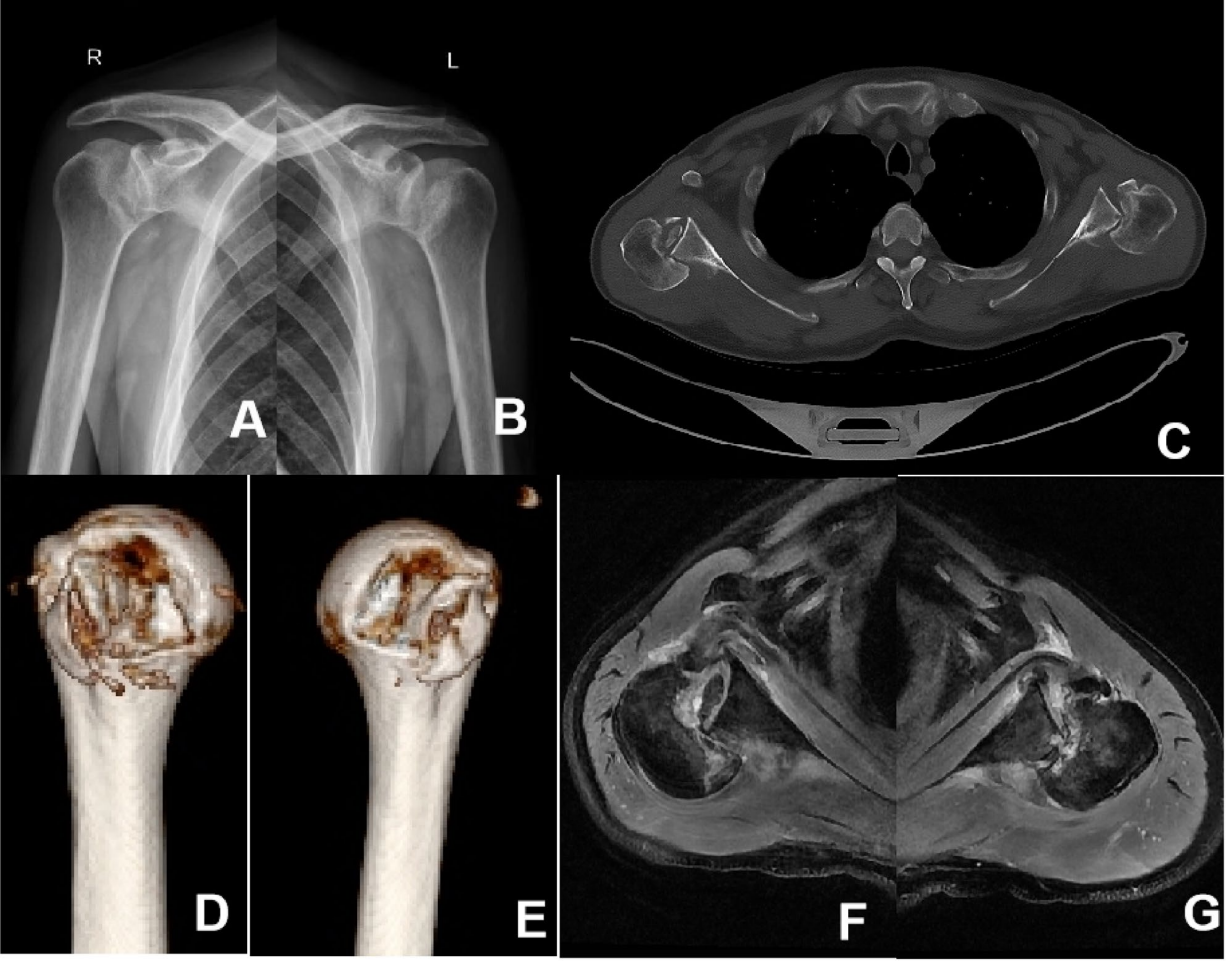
Preoperative images of the patient A AP view of the right shoulder; B AP view of the left shoulder; C Bilateral CT scans; D Three-dimensional reconstruction of the right humeral head; E Three-dimensional reconstruction of the left humeral head; F Right shoulder MRI scan; G Left shoulder MRI scan
